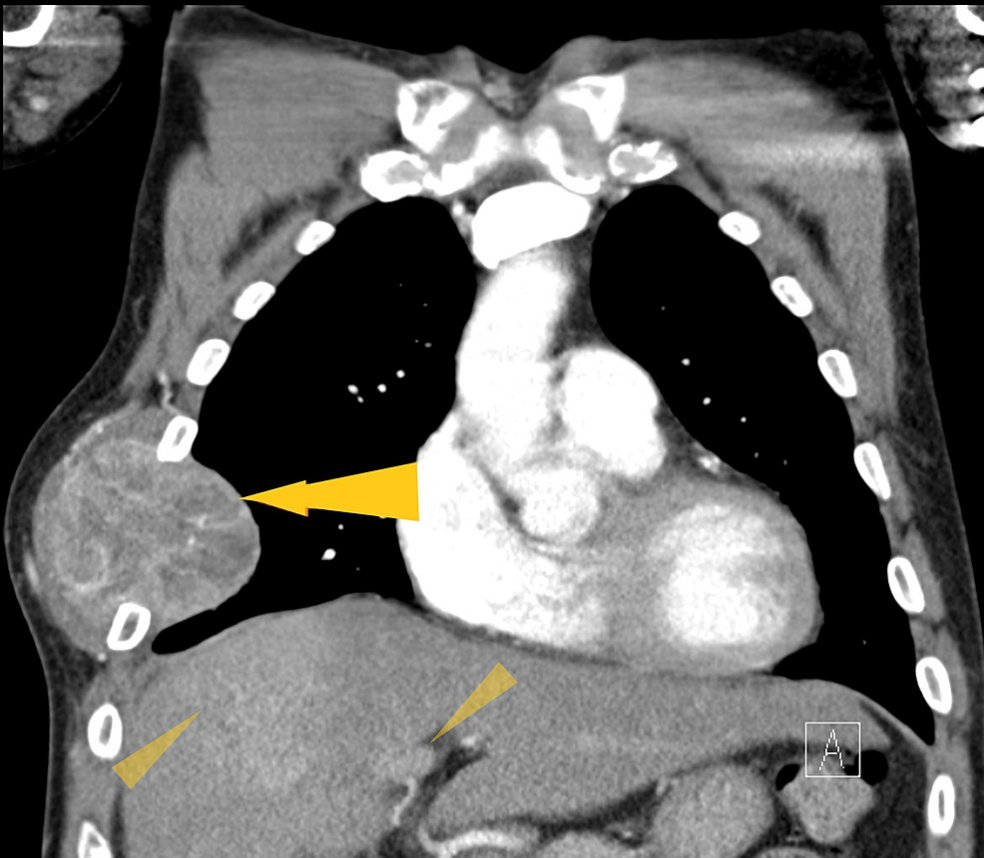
Computed tomography of the chest, with contrast, shows a heterogeneously enhanced soft tissue mass arising from the right anterolateral sixth rib (yellow arrow), which was eroded and replaced by a portion of the mass that appeared extrapleural. Multiple ill-defined, hyper-attenuating foci in the liver are present, including a larger one measuring 2×1.2 cm in the right lobe and one measuring 2.8 cm×1.4 cm in the gastrohepatic lymph node (yellow triangles).
